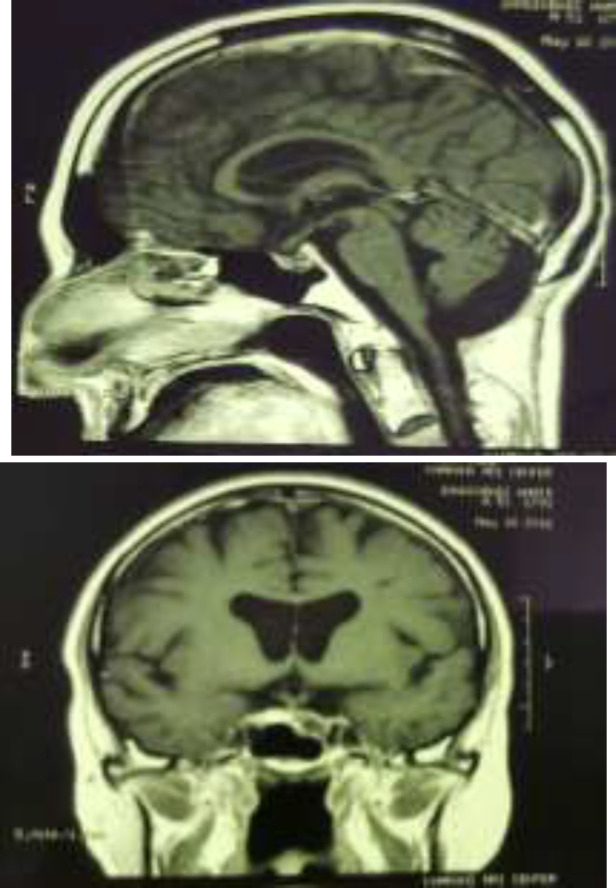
Postoperative T1 coronal and sagittal MRI after total mass resection in the second surgery
